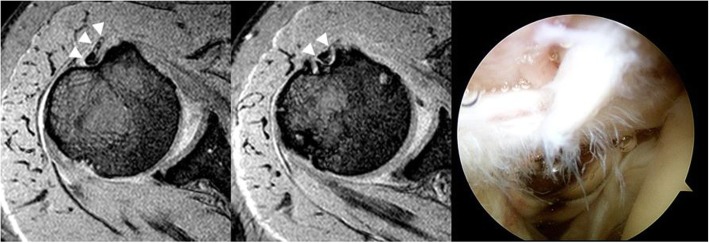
A T2-weighted axial MRI showing severe contour irregularity of the tendon at the bicipital groove (white arrowhead). This patient’s MRI revealed a complex tear involving nearly the full width of the long head of the biceps tendon in the bicipital groove (Type V)
